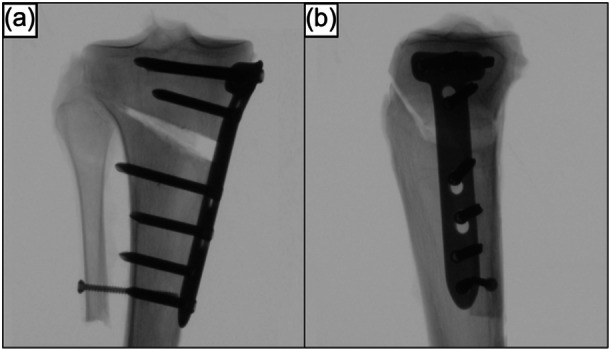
Anteroposterior (a) and mediolateral (b) radiographs of a right proximal tibia after a biplanar, medial open wedge high tibial osteotomy fixed with a locking compression plate. Care was taken to preserve an intact lateral hinge.
